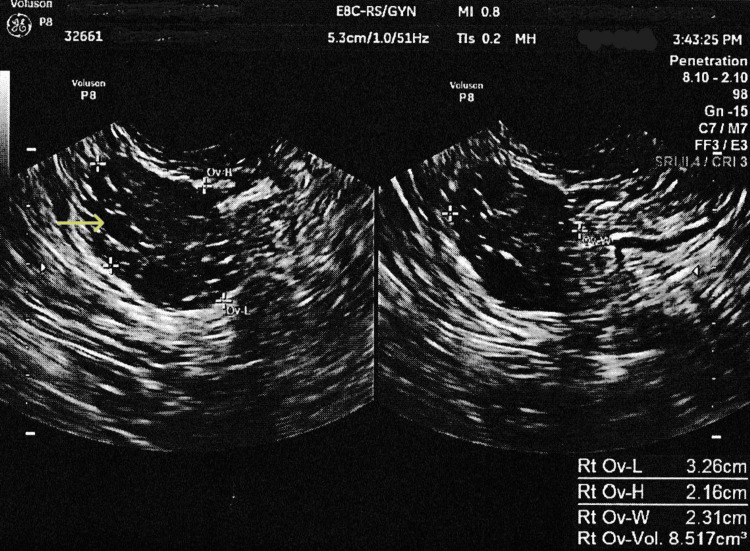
Pelvic transvaginal ultrasound showing a polycystic right ovary
